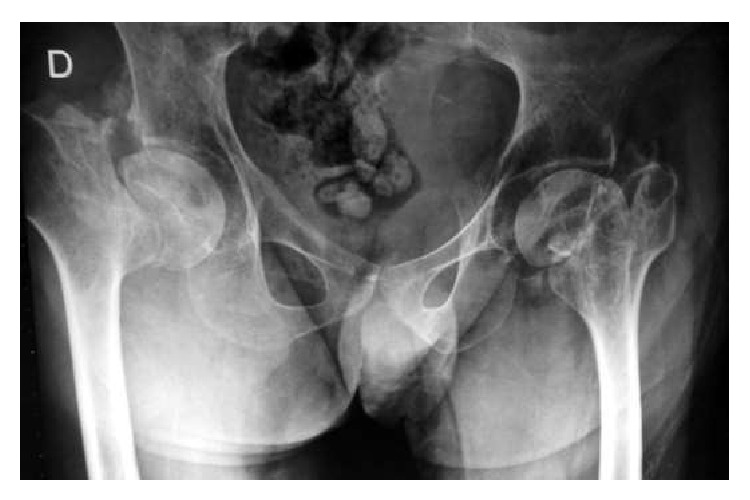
Anteroposterior both-hip radiograph showing bilateral Garden IV femoral neck fracture.
